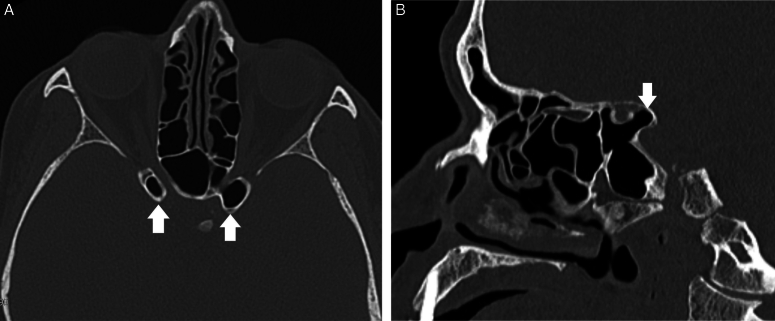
Fig. 6A is an axial CT image of PNS at the level of optic nerves. Figure 6B is mid sagittal CT image. Pneumatized anterior clinoid process is seen bilaterally in both planes (white arrows).
